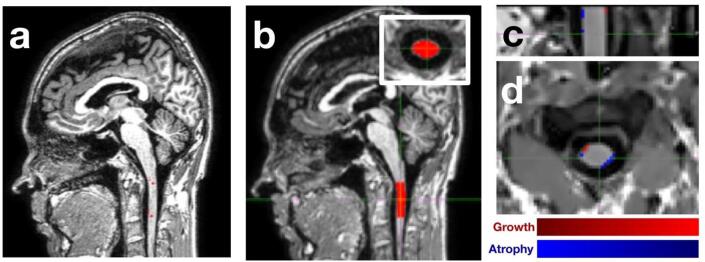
Brain MRI processing using SCT and GBSI. Reference points were manually set at C1 and C2 on sagittal brain scans (a). Then, masks of C1-2 (b) levels were obtained from spinal cord images acquired at each time point using the *DeepSeg* tool within the SCT (version 4.0) (sagittal plane is presented, with axial plane in the inset). The probabilistic boundary-shift region-of-interest was then adaptively estimated from baseline and follow-up spinal cord segmentations and the GBSI integral was ultimately computed for C1-2 (c for sagittal plane, d for axial plane). CSA: cross-sectional spinal cord area; GBSI: generalised boundary-shift integral; MRI: magnetic resonance imaging; SCT: spinal cord toolbox.
